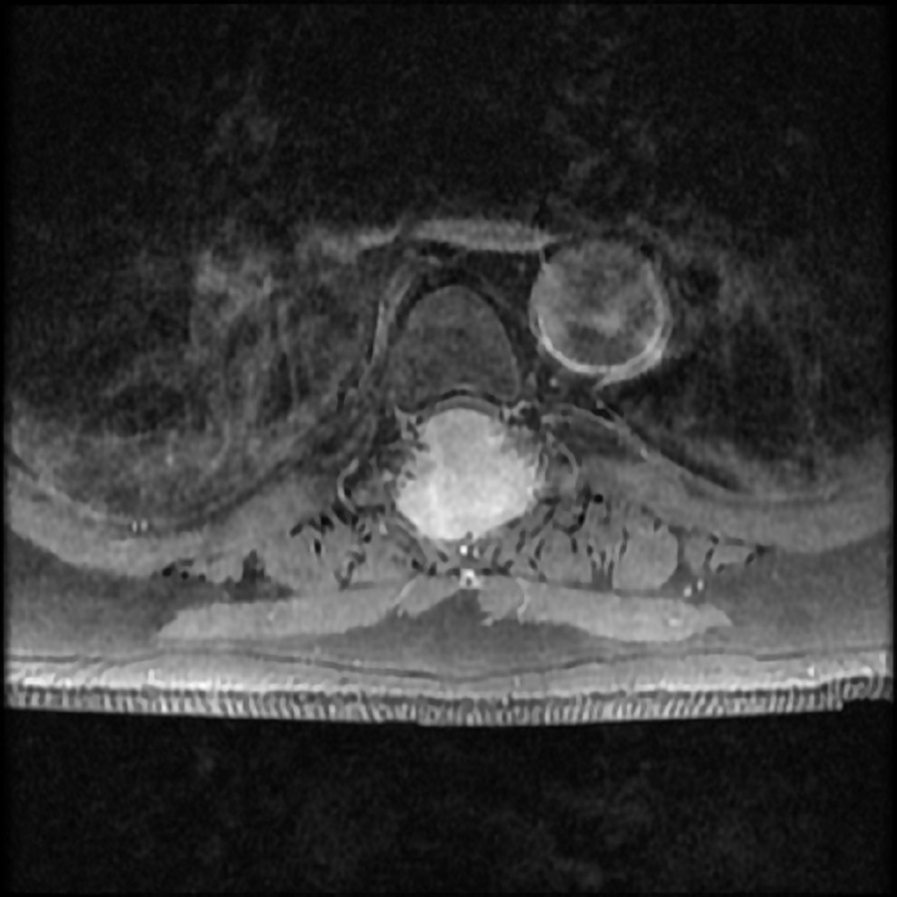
T1 axial images with contrast MRI of the thoracic spine at T1 with contrast axial images revealed an approximately 2.9 x 3.8 x 3.7 cm extradural midline mass centered in the posterior elements of T5. The mass was extending into the spinal canal with severe thoracic cord compression. There was increased signal within the spinal cord.
